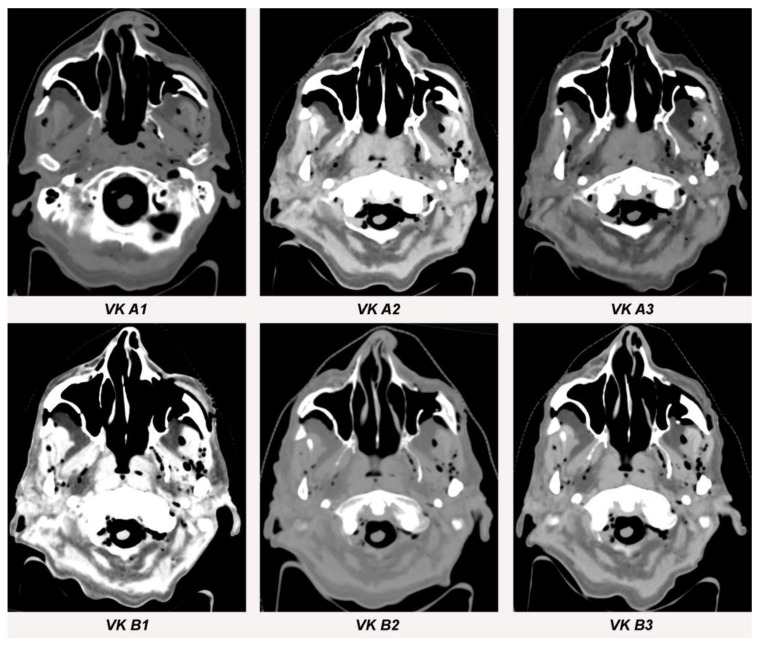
Streak artifacts VK A1, VK A2, VK A3, VK B1, VK B2 and VK B3 in CT images in axial orientation versus control. Artifacts of each implant were measured at three different anatomical landmarks: (1) the zygomatic soft tissue, (2) the temporal muscle and (3) the parotid gland.
